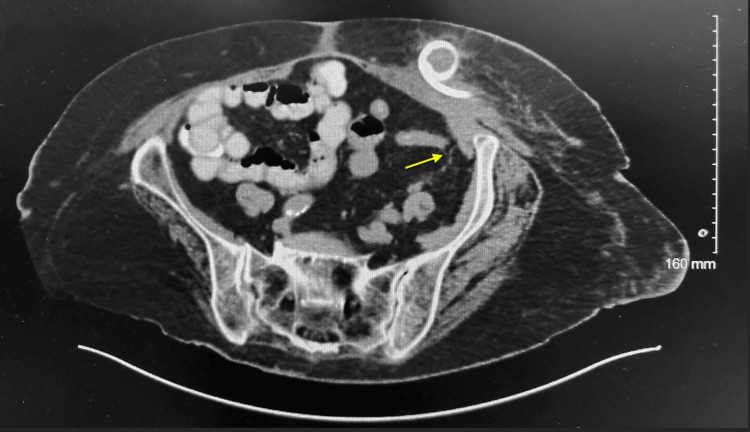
CT scan showing closely opposed bowel in the LLQ raising suspicion for an enterocutaneous fistula (arrow) LLQ: Lower left quadrant
